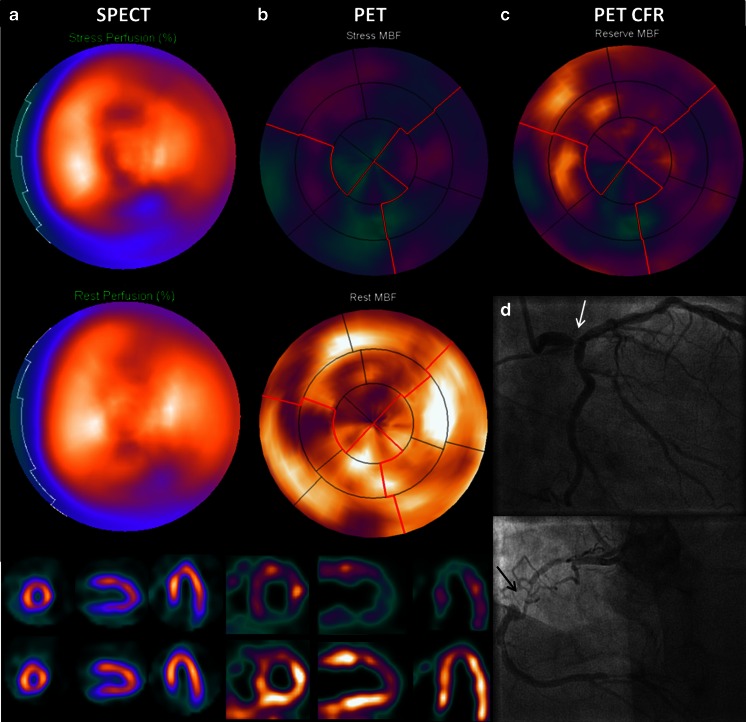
Balanced ischemia on SPECT. Example of a (nearly) normal SPECT (a) and severely reduced perfusion on positron emission tomography (PET) (b, c) in case of a significant left main stenosis (*white arrow*) and a chronic total occlusion of the RCA (*black arrow*) on invasive coronary angiography (d). SPECT is hampered by the relative nature of the images, whereas quantification with PET allows for detection of diffusely balanced ischemia. Abbreviations as in Figs. 2 and 3
